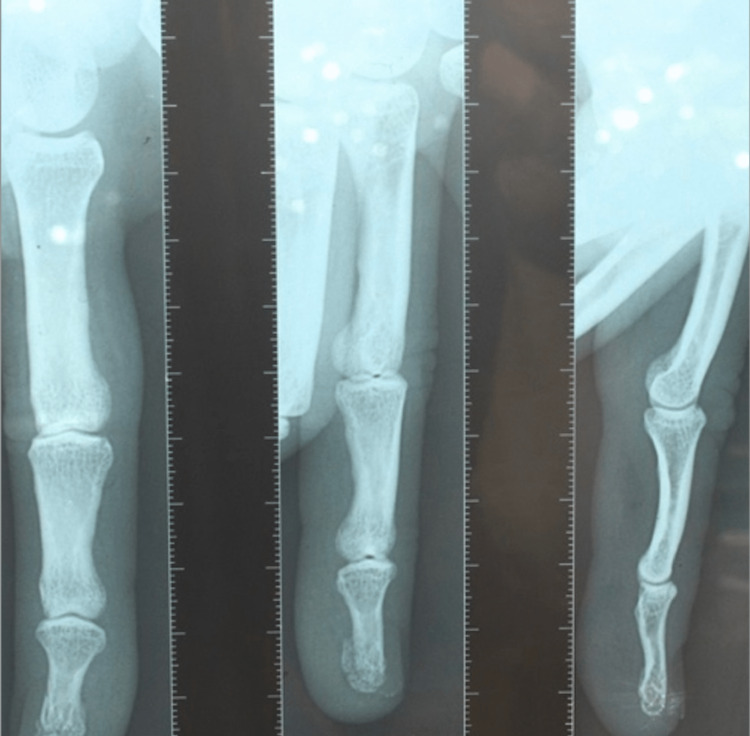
Standard radiographs of the 3rd finger, anteroposterior and lateral views, without anomalies
